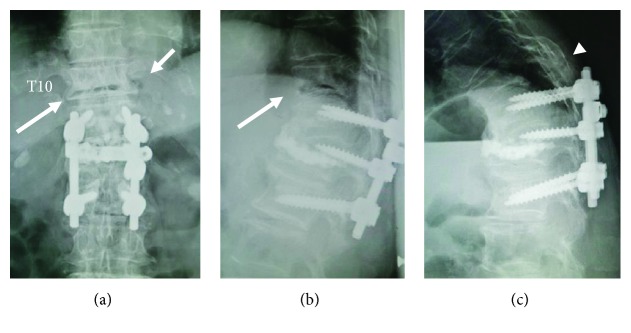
Preoperative thoracolumbar spine radiographs in the supine (a, b) and sitting (c) positions, showing intravertebral vacuum cleft (long arrows), extravertebral gas (short arrow), and the fracture line of T10 extending to posterior fusion mass bone (arrow head) grafted 8 years ago.
